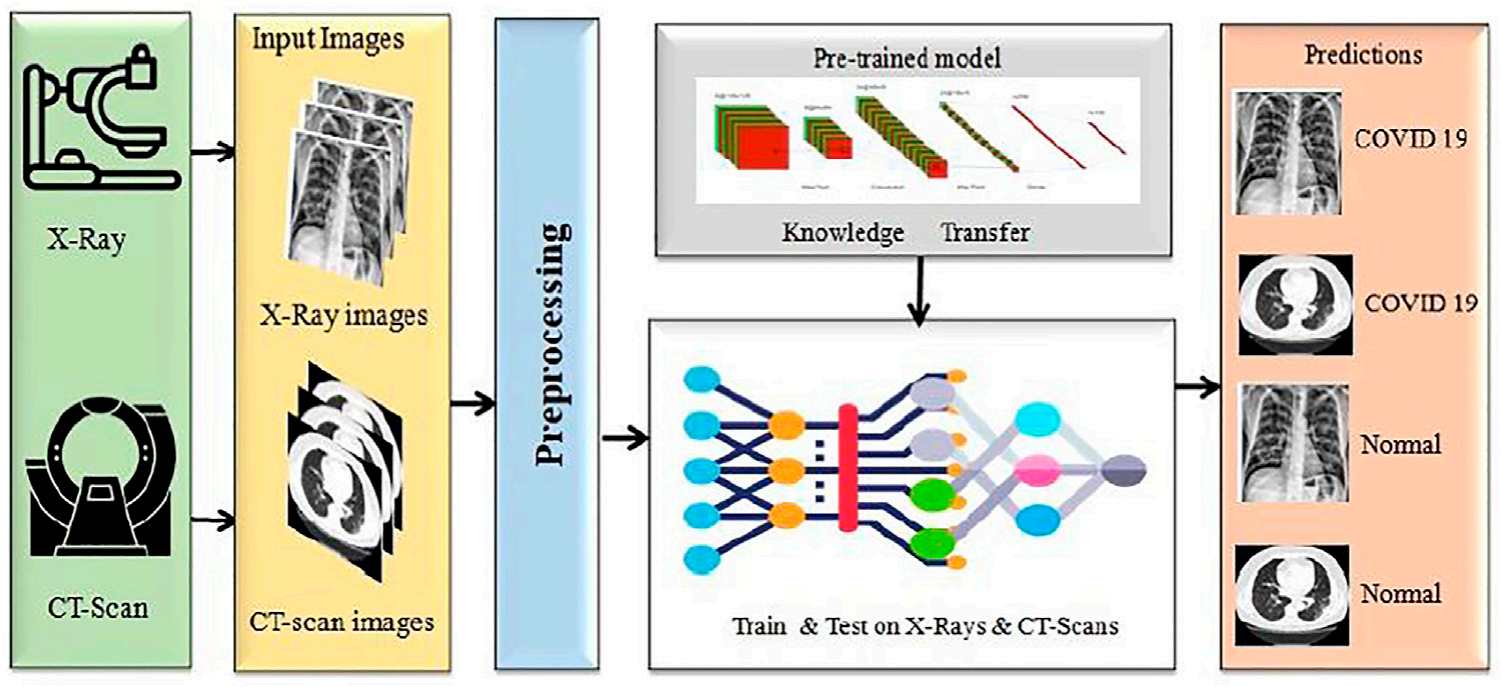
The proposed COVID-19 detection framework using both X-ray and CT-scan.
